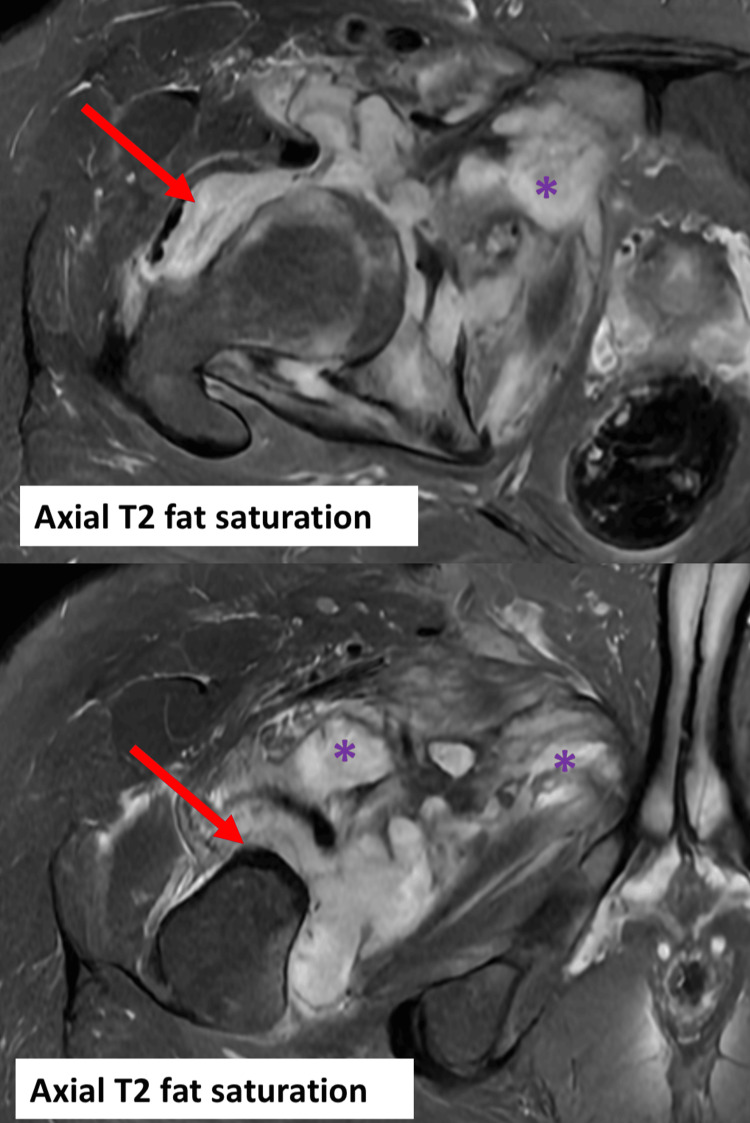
MRI of the right hip with axial T2 fat saturation. Right-sided joint effusion (red arrows) and multifocal intramuscular collections (purple asterisks) tracking into the medial thigh compartment and medial pelvis.
